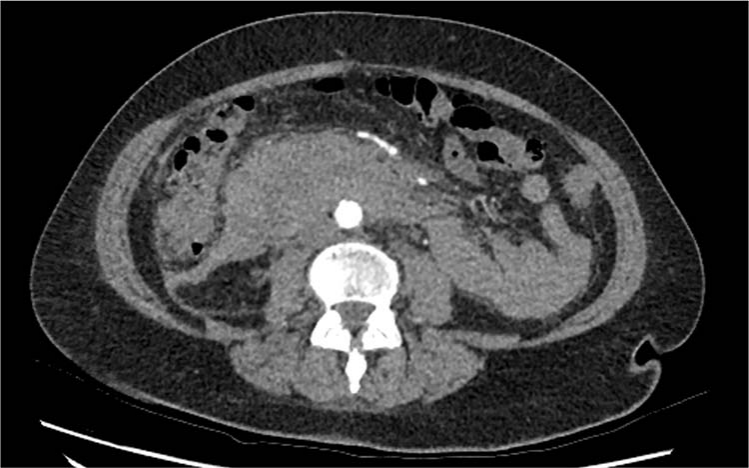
CT triple phase, axial view. Stable periduodenal hematoma, no acute hemorrhage or vascular abnormality identified.
